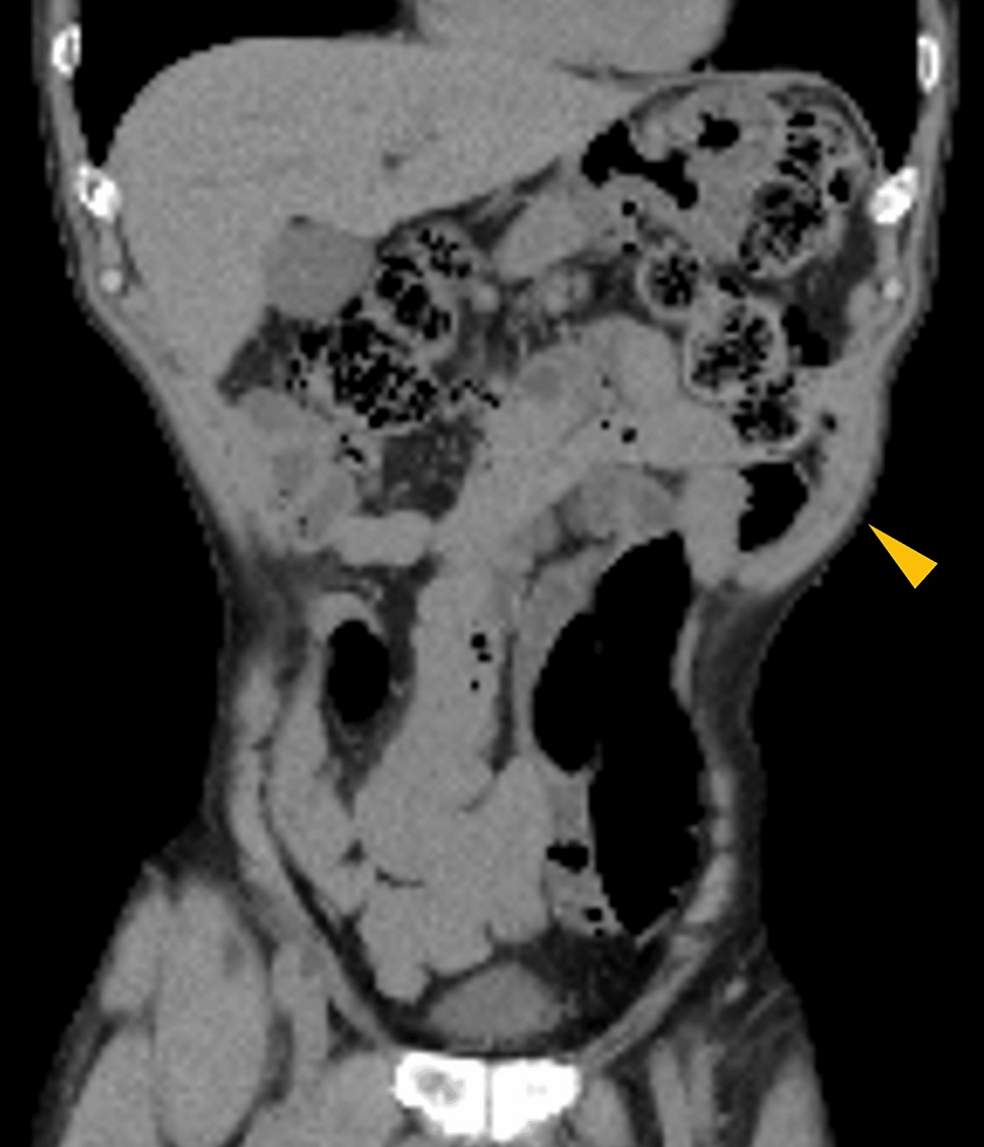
Plain abdominal CT imaging of the patient. The plain abdominal CT imaging revealed muscle relaxation in the left upper abdominal bulge area with no obvious hernia sac or hernia contents (arrow).
